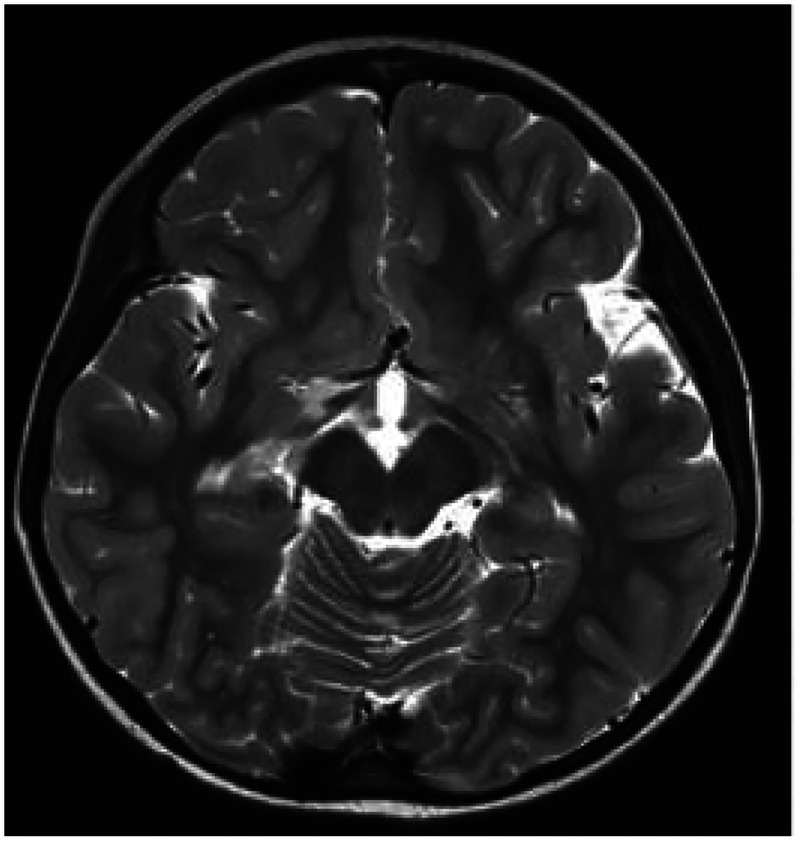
Pre-rupture MRI demonstrates an AComA aneurysm with associated congenital midline anomalies.
